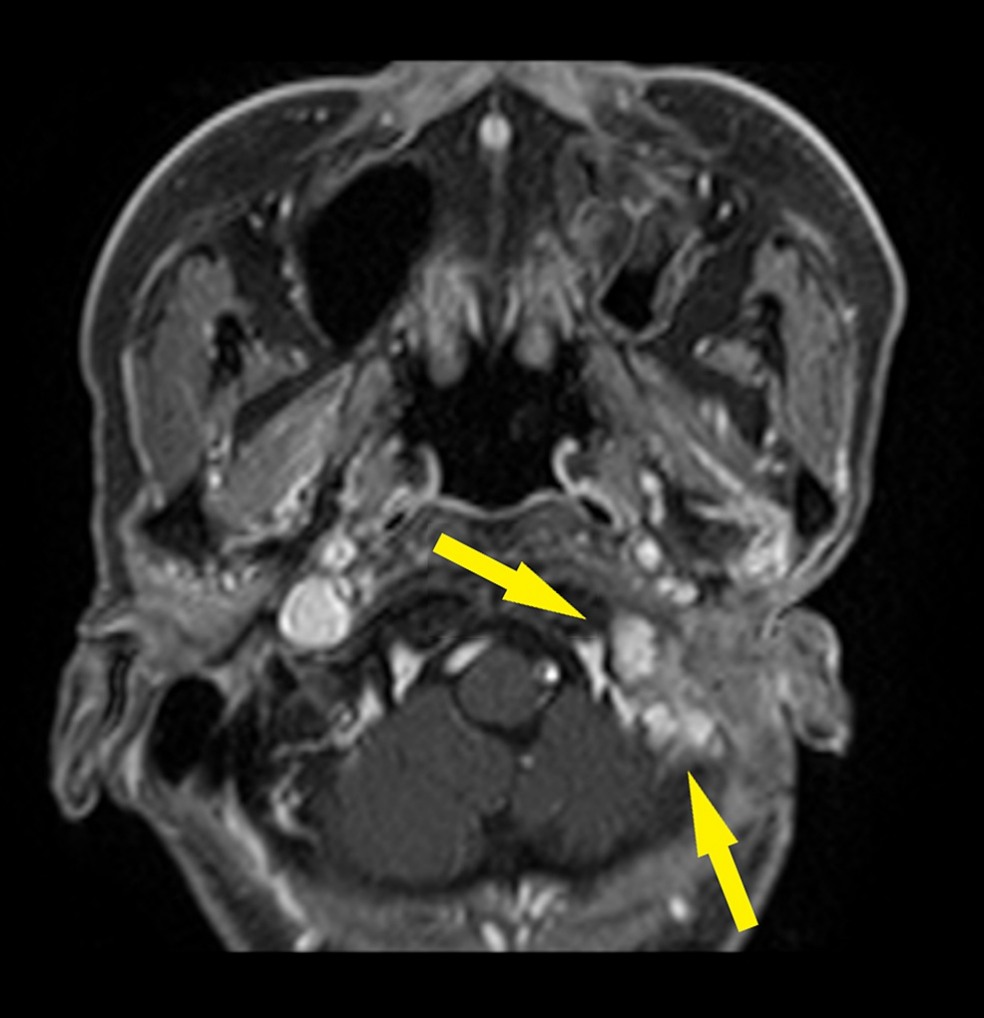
Second recurrence in March 2019 Gadolinium-enhanced fat-saturated T1-weighted image Yellow arrows: a lesion suggestive of recurrence
